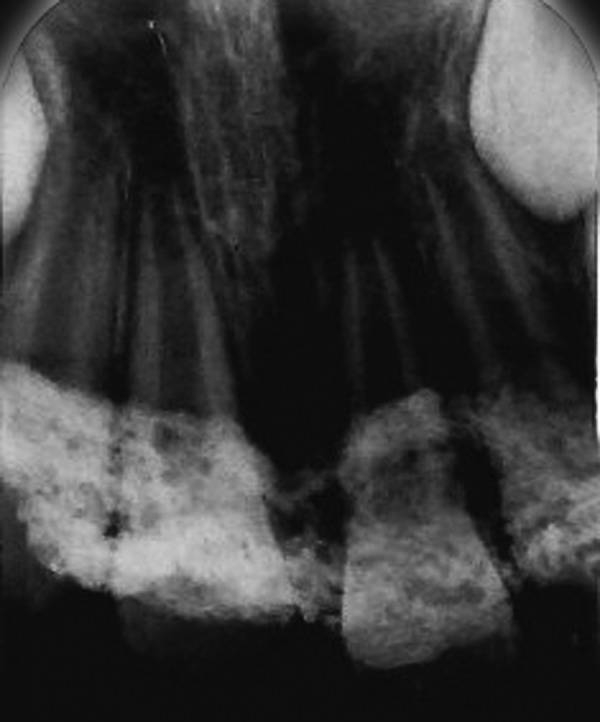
Preoperative intraoral periapical radiograph (case 4)
